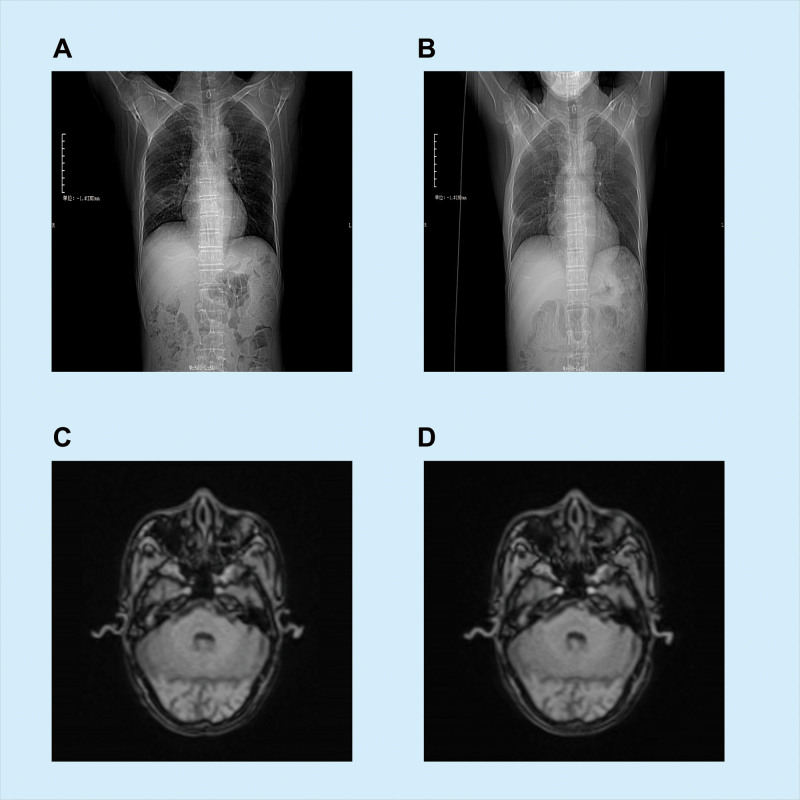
Radiological examination before and after treatment. (A) Chest CT before treatment. (B) Chest CT after treatment. (C) Pretreatment MRI examinations of the patient. (D) Posttreatment MRI examinations of the patient. CT = computed tomography, MRI = magnetic resonance imaging.
